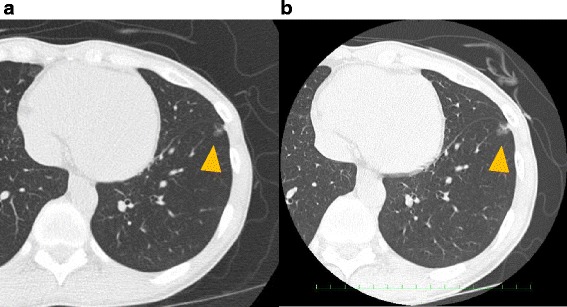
Thin-section CT showed a GGN with a small solid component in the left lower lung. CT during a medical checkup showed a nodule. Follow-up CT 2 years after the medical checkup showed that the nodule had grown slightly. Yellow allowheads indicate the location of tumor
